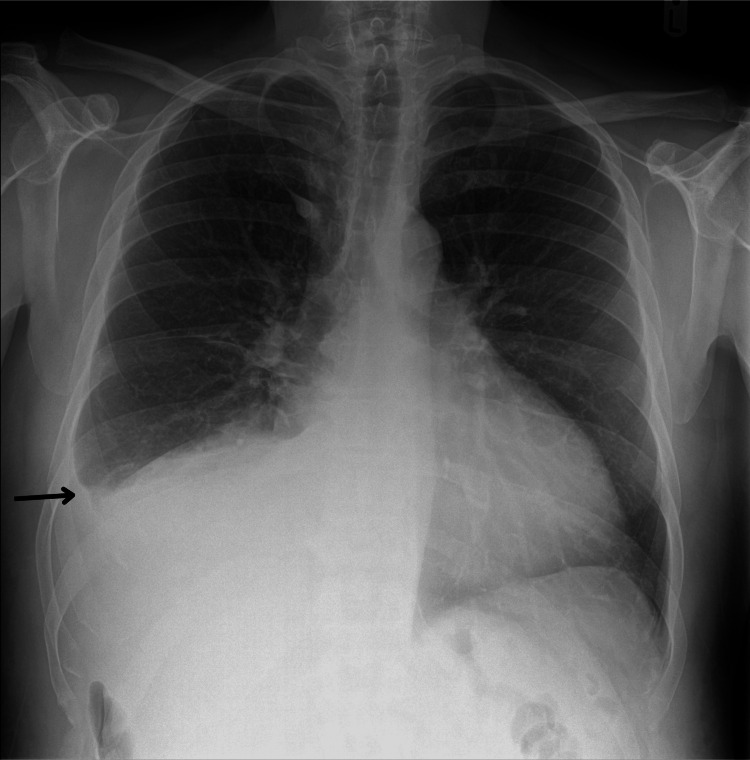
PA radiograph of the thorax on admission. The PA radiograph of the thorax shows blunting (arrow) of the right costophrenic angle. PA: posteroanterior.
